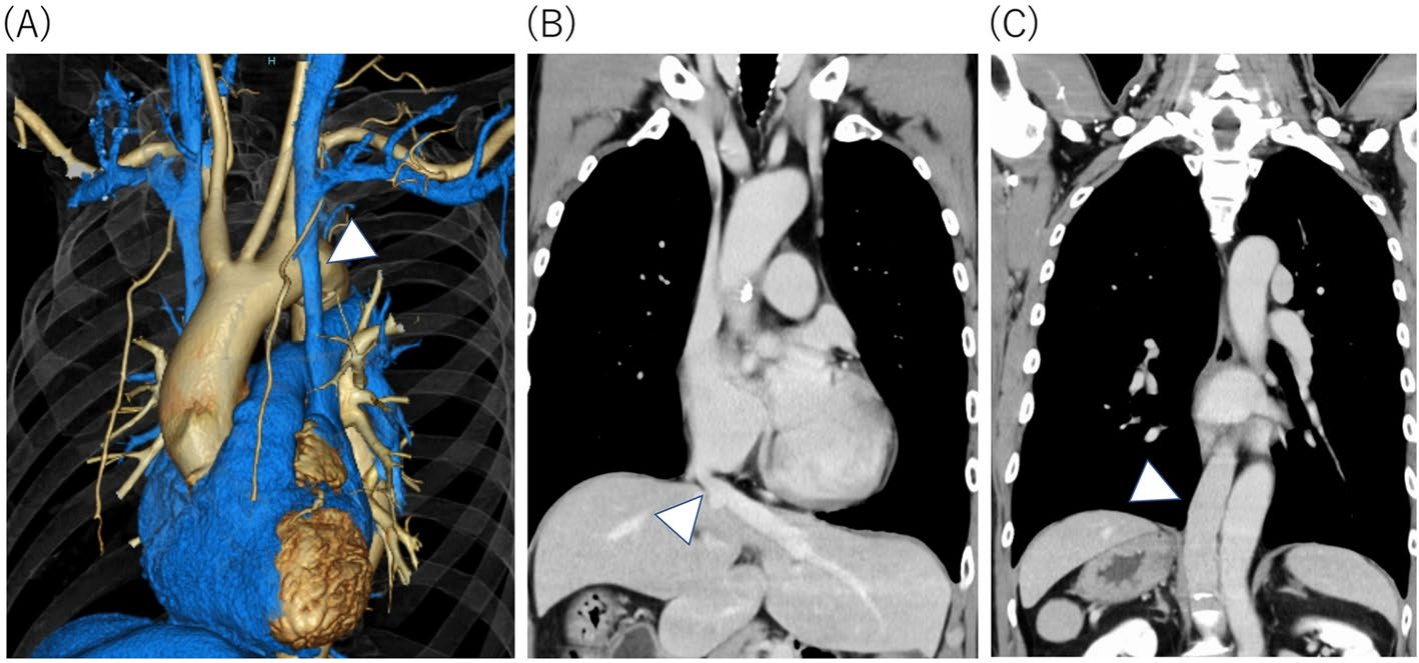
(A) Contrast-enhanced computed tomography showed the presence of the PLSVC (triangular arrow) that flows into the right atrium. (B) The hepatic vein (triangular arrow) flows directly into the right atrium. (C) The inferior vena cava (triangular arrow) enters the thoracic cavity next to the descending aorta and flows into the parazygous vein and PLSVC
